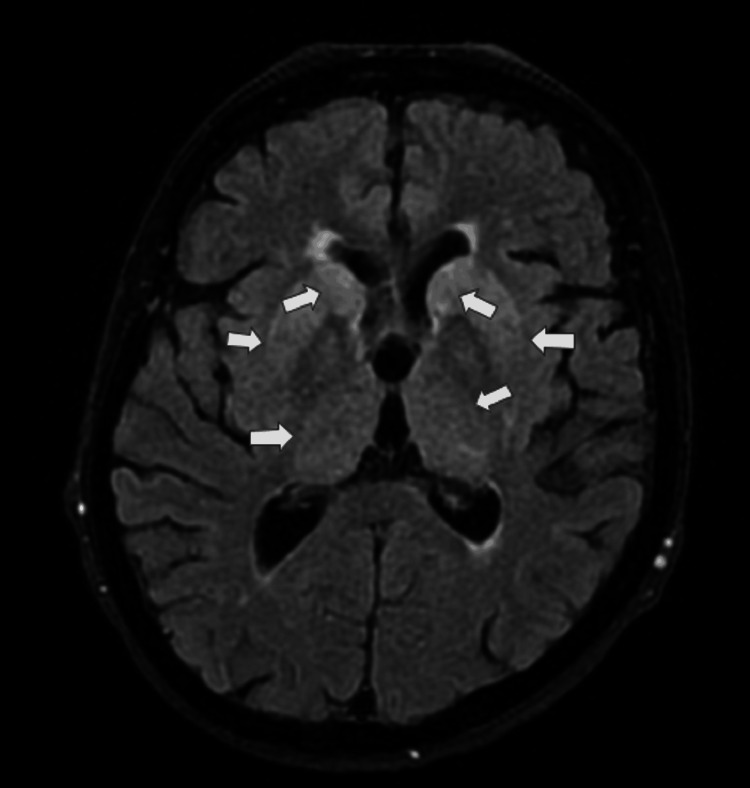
Axial FLAIR MRI showing bilateral hyperintense signal changes involving the caudate nuclei, lentiform nuclei, and thalami nuclei (white arrows). These deep grey matter abnormalities are suggestive of Creutzfeldt-Jakob disease FLAIR: fluid-attenuated inversion recovery; MRI: magnetic resonance imaging
